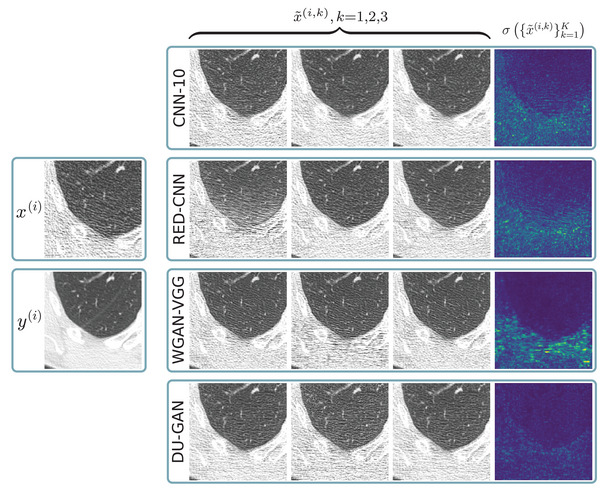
Invariances for a random crop i from the test set. Shown are low‐dose image $x^{\left(i\right)}$, high dose image $y^{\left(i\right)}$ and three reconstructed invariances ${\tilde{x}}^{\left(i,k\right)}$ for each of the four denoising methods. We also show standard deviations $\sigma \left({\left\{{\tilde{x}}^{\left(i,k\right)}\right\}}_{i=1}^{K}\right)$ over K=100 invariances. For CT Images: C=−600HU, W=1500HU, for standard deviations: C=0HU, W=400HU.
